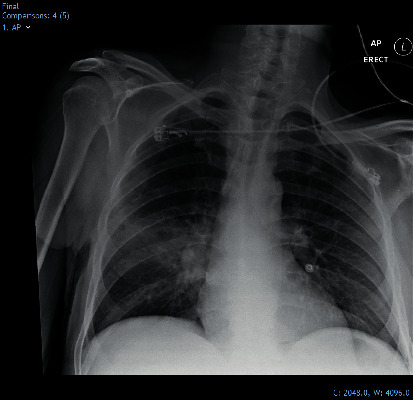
Chest radiograph on admission demonstrating right midzone consolidation.
